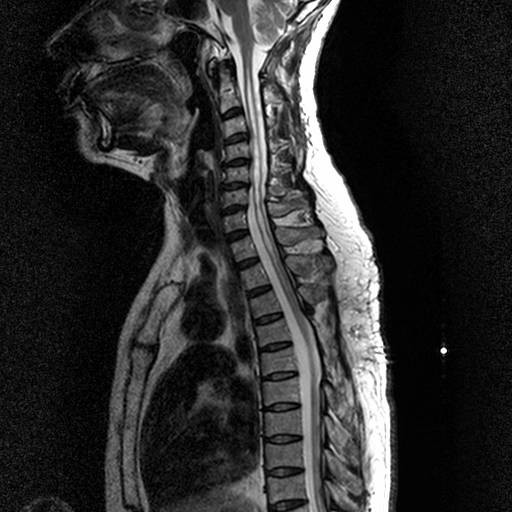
Post-operative cervical spine MRI studies demonstrate substantial diminution in size of the syrinx on T2-weighted image. The central canal was reconstructed using a tube.
